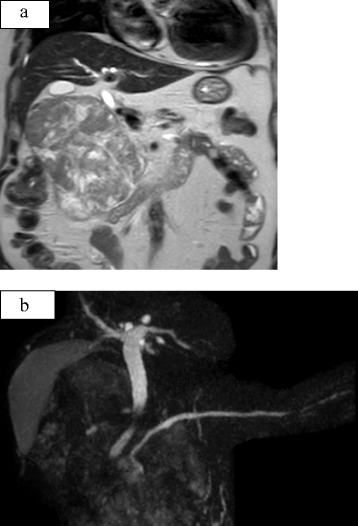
Dynamic magnetic resonance image showing a 13.5 × 12 × 8 cm hypervascular tumor containing necrotic areas in the pancreatic head (a). Magnetic resonance cholangiopancreatography showing no evidence of involvement of pancreatobiliary trees (b)
